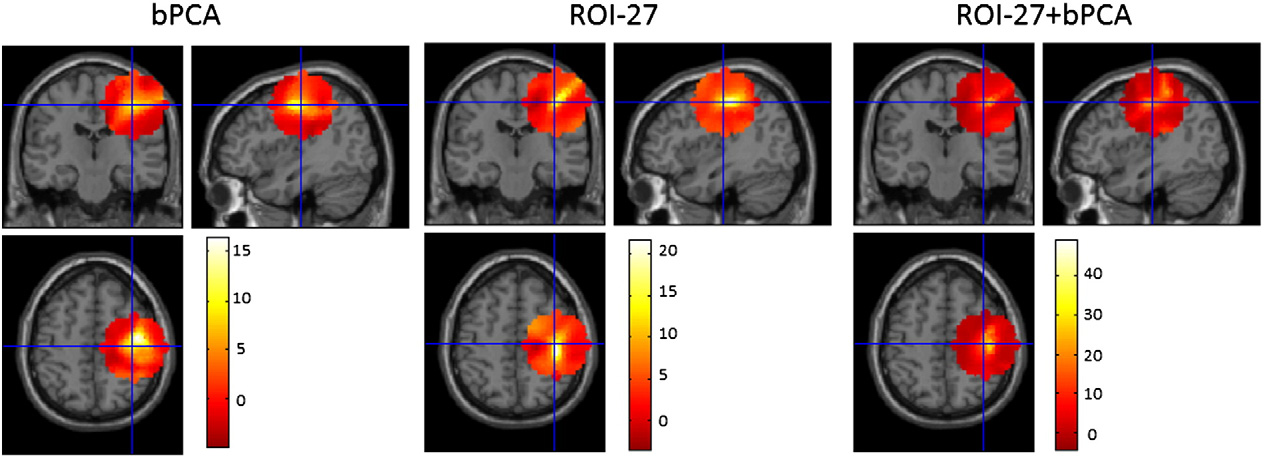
T-images contrasting the source ‘on’ and source ‘off’ conditions, superimposed on a canonical T1-weighted MRI, for a 30 mm spherical volume surrounding the source of interest (at MNI coordinates 40–20 50) are displayed. Coronal, sagittal and axial sections through the images are shown with the crosshairs centred on the source of interest. Note that colour bars are shown separately for each image. Combining the ROI projection with bPCA results in the highest t values in the vicinity of the source of interest.
